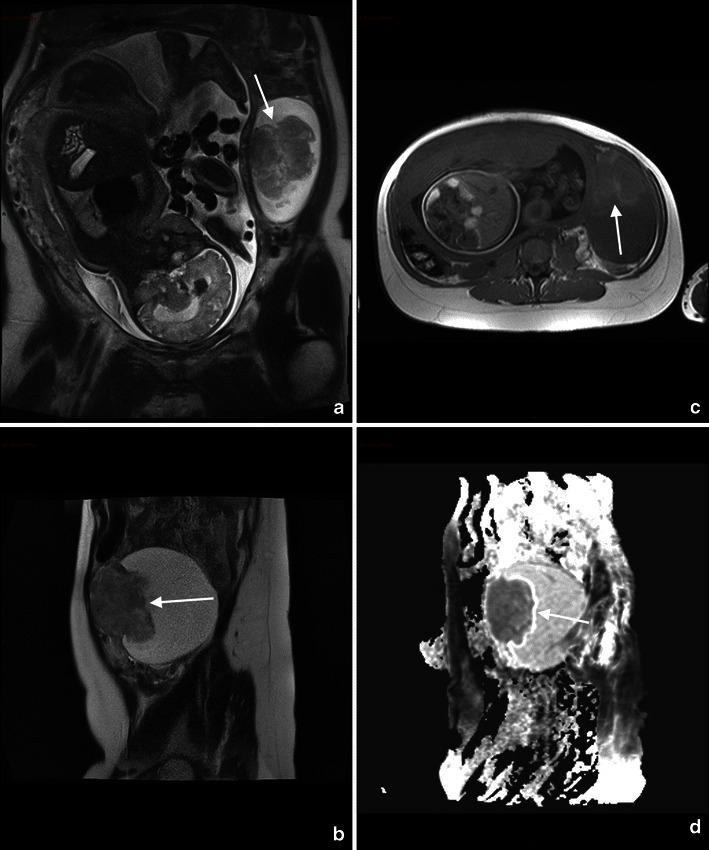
Coronal (a) and sagittal (b) T2-weighted MR images show a complex mass, with fluid and solid components on the left ovary. Haemorrhagic areas are seen on T1-weighted sequence (c). Sagittal DWI image shows reduction of diffusion in relationship to the high cellularity of the solid component of the mass (d). Cystoadenocarcinoma was confirmed at surgery
